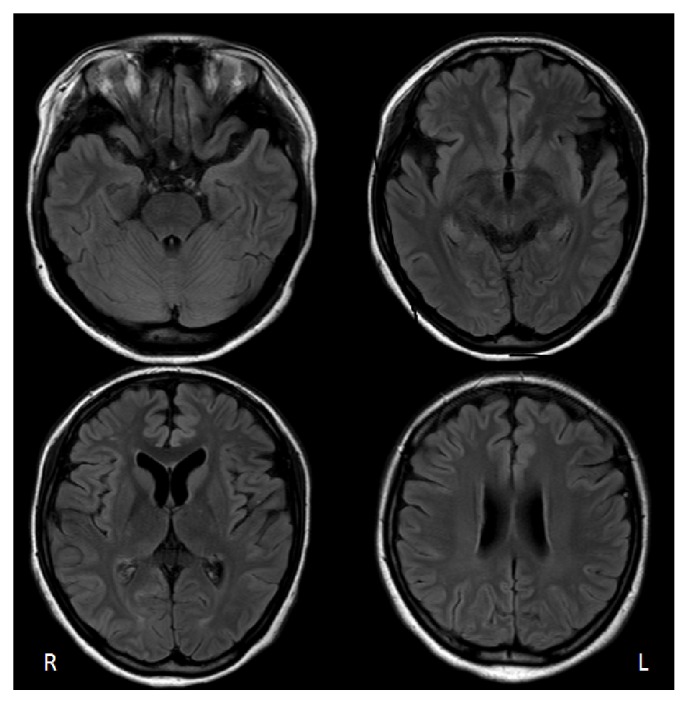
Axial fluid-attenuated inversion recovery (FLAIR) images of the brain two months after admission. Note the slight high intensities in the bilateral occipital cortices and subcortical white matter, which were somewhat prominent on the right side, as well as small subcortical high intensities in the right frontal lobe. However, there were no signal abnormalities in the medial temporal areas.
